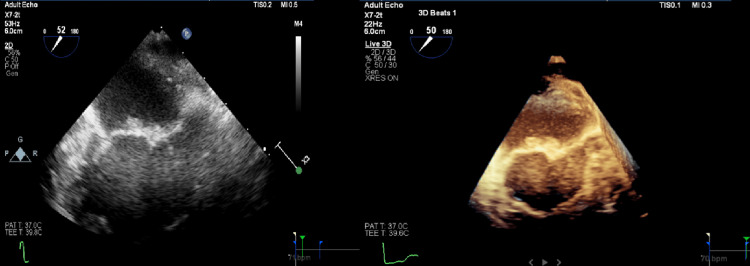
Transesophageal echocardiography demonstrating multiple large vegetations on the anterior and posterior leaflets of the mitral valve.
